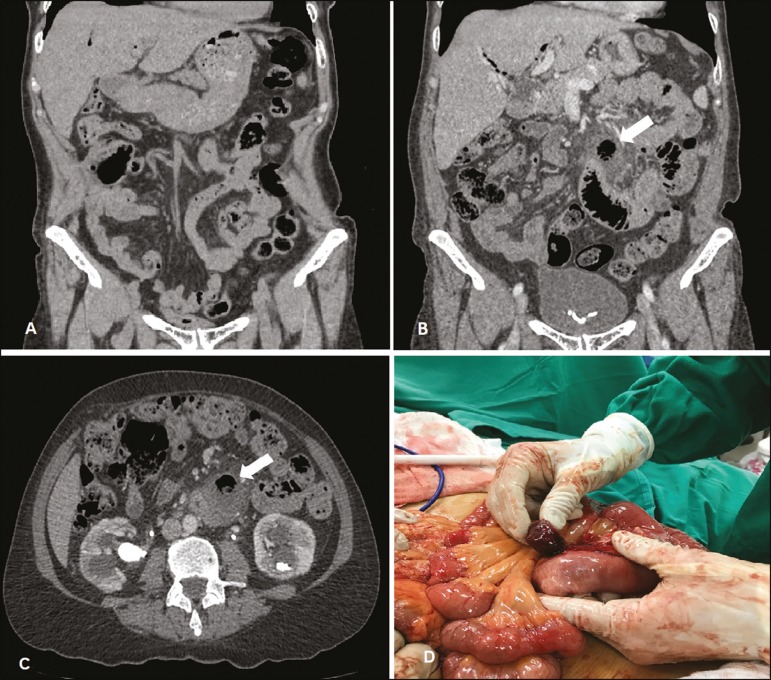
Coronal CT (A), acquired six months prior, in which no
